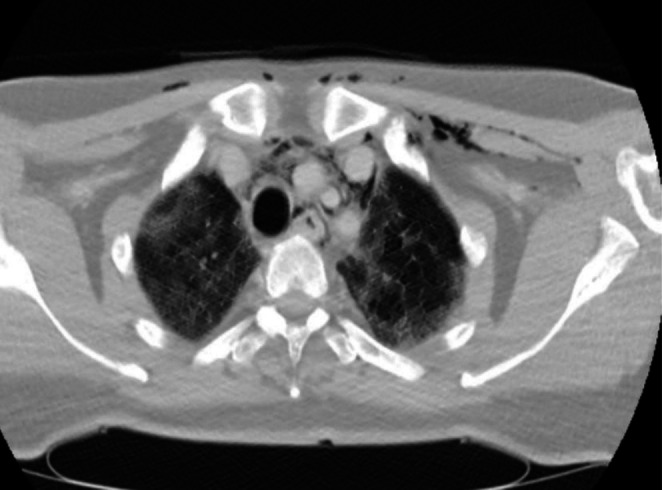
Non‐contrast CT scan of the chest: A Single representative slice image of a non‐contrast chest CT scan on Lung window at the level of T3 (above the arch of aorta) demonstrates bilateral diffuse mixed consolidation and GGO with interlobular smooth septal thickenings (crazy paving appearance). It also shows free air in the mediastinum surrounding the major vessels and the airway (pneumomediastinum) as well subcutaneous emphysema over anterior chest wall and lower neck more exuberant on the left side.
